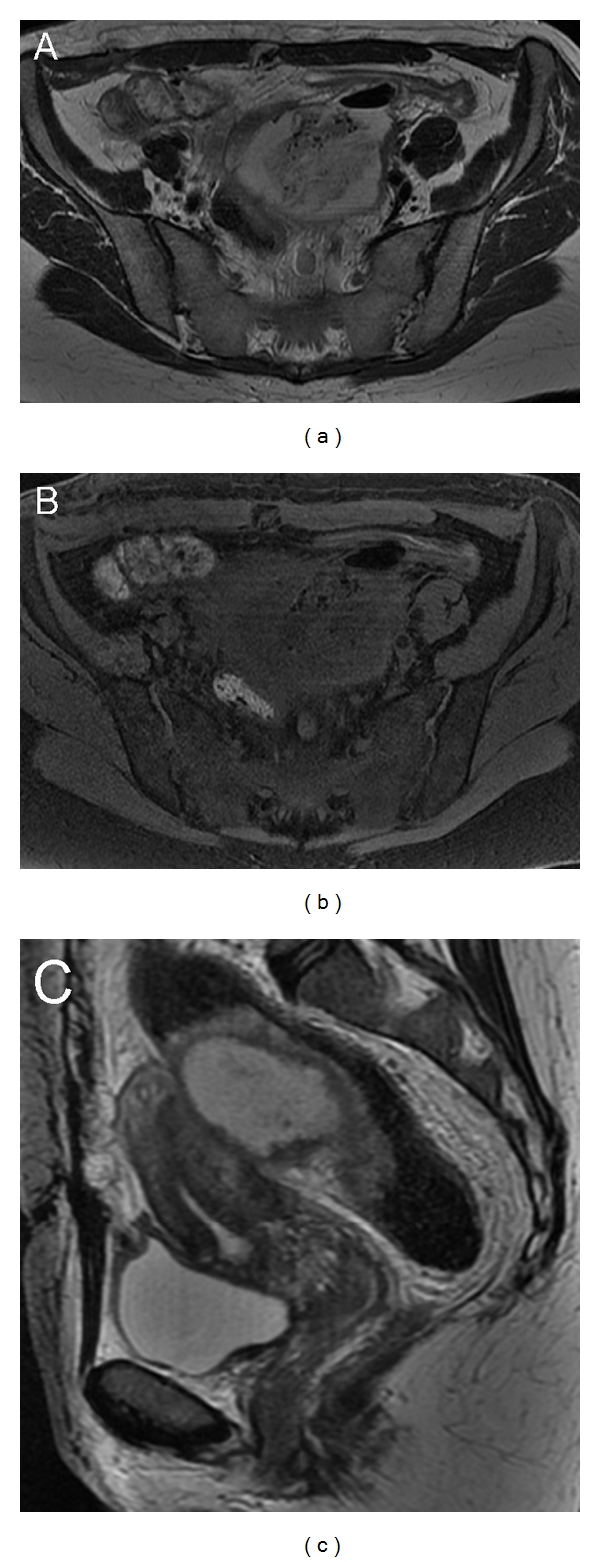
Pelvic inflammatory disease. Left recurrent tuboovarian abscess. Axial T2-weighted image (a) shows a thick wall, complex, heterogeneously hyperintense, fluid-containing adnexal mass, with internal debris and gas bubbles, that represents the most specific sign of an abscess. On axial T1-weighted with fat suppression image (b) there is no signs of hemorrhagic contents within the mass. The sagittal T2-weighted image (c) demonstrates the involvement of bowel loop characterized by the thickening of the anterior rectal wall.
